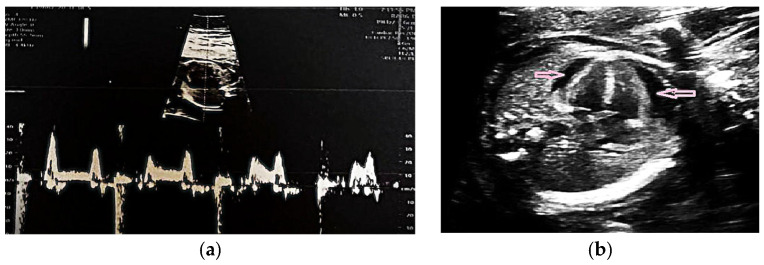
(a) Fetal echocardiography at 20 weeks of gestation with CAVB with a heart rate of 64 beats per minute (bpm); (b) Fetal echocardiography at 24 weeks of gestation showed a heart rate of 59 bpm, cardiomegaly and pericardial effusion (pink arrows).
